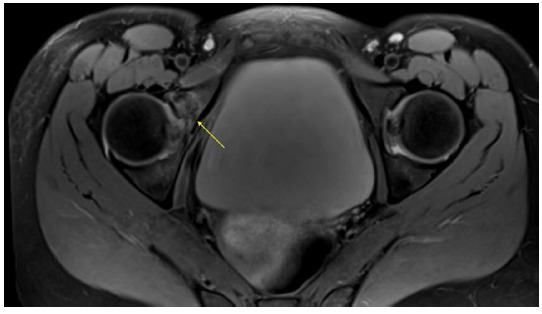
Case 2: MRI scan of the right hip on the day of injury demonstrating a fracture of the anterior column
